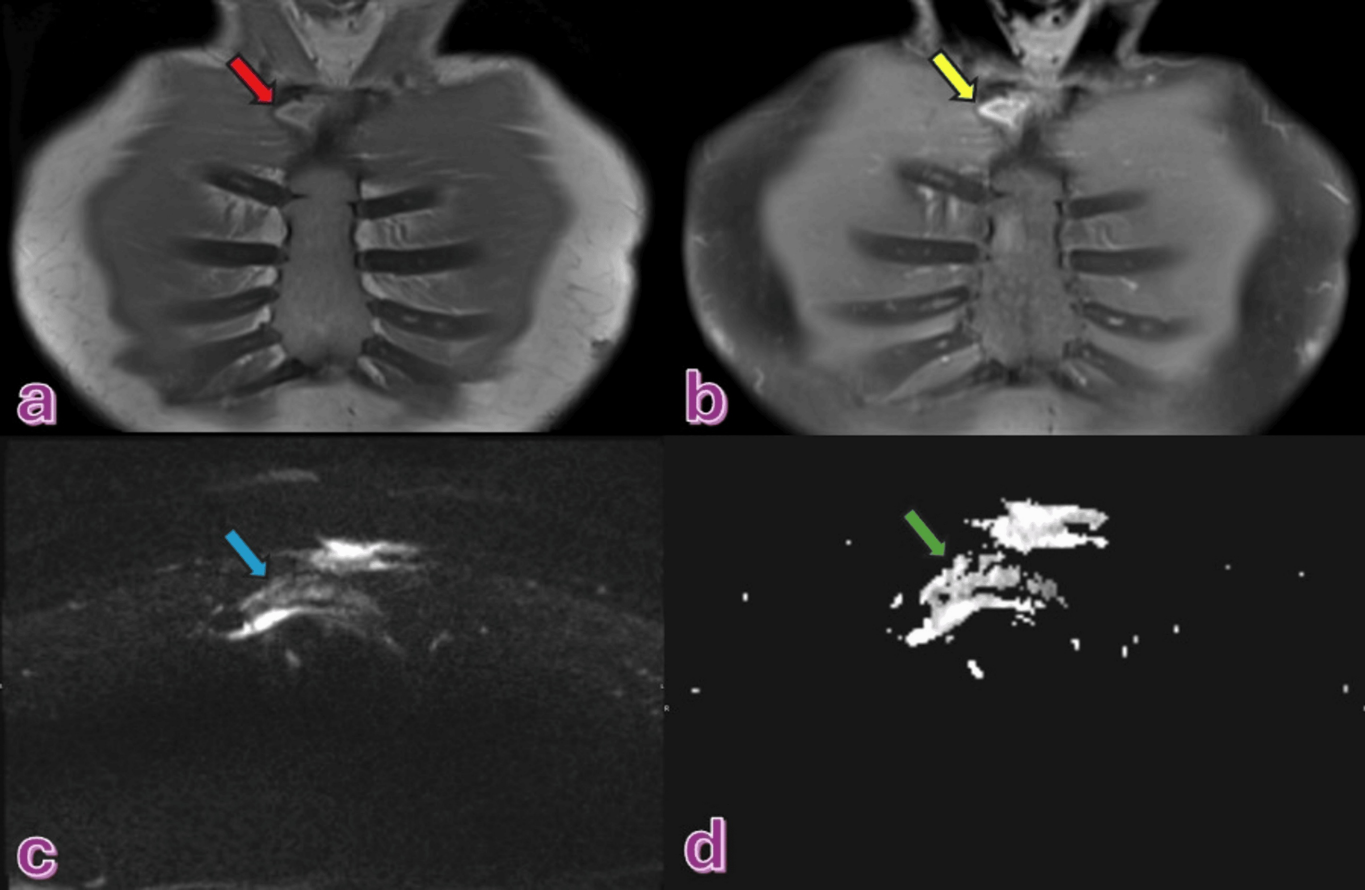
A well-defined slightly heterogenous lesion with intermediate signal noted in the lateral right aspect of the manubrium sternum in T1WI (red arrow) (a), the lesion demonstrates post-contrast enhancement seen in the post-contrast T1W1 (yellow arrow) (b). It also shows partial diffusion restriction in DWI (c) and ADC (d) (blue and green arrow)
